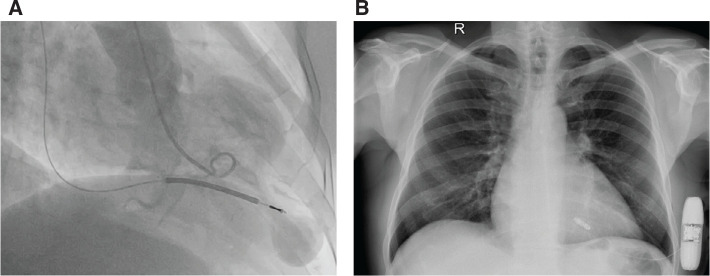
A: Left ventriculography in systole, revealing an apical aneurysm. B: A chest X-ray after implantation of a leadless pacemaker and a subcutaneous defibrillator.
